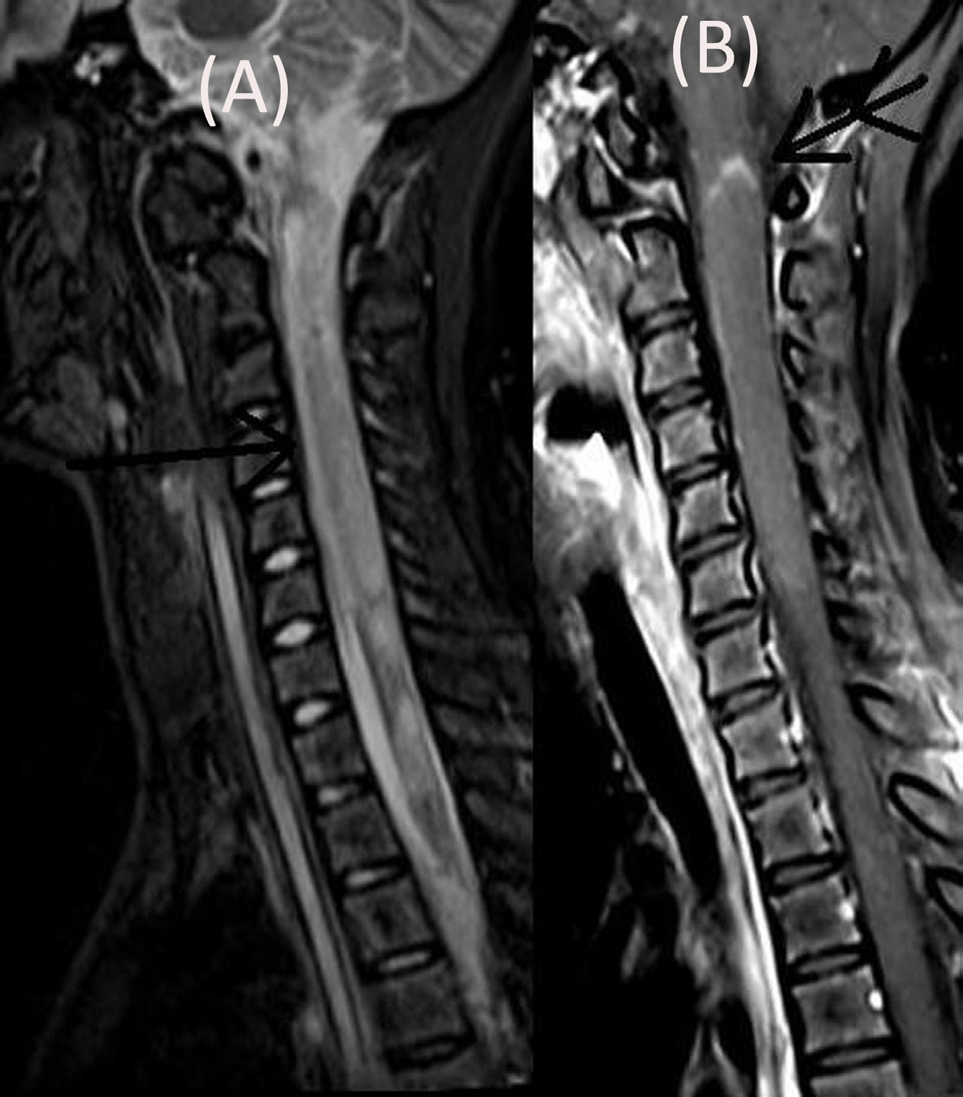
Cervical MRI showing long segment T2 hyperintensity (arrow) (A) patchily enhancing in cervical cord (double arrow) and dorsal cord (B).
